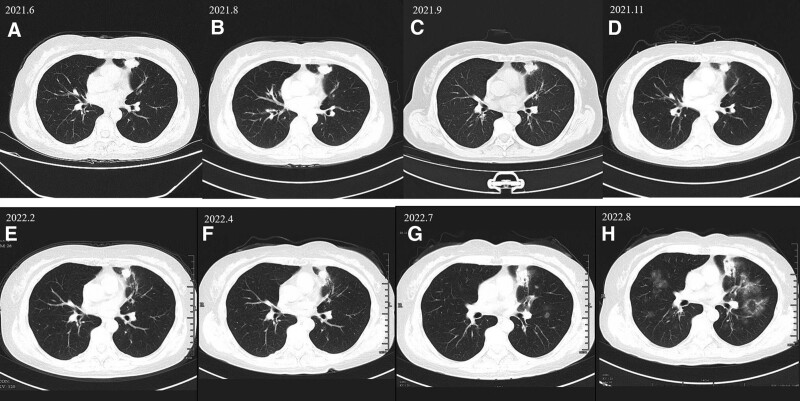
Results of chest CT scans before and after ICI treatment in a patient with cervical adenocarcinoma. (A) 2021.6.16. Tumor size: 25 × 20 mm. (B) 2021.8.3. The tumor measured 24 × 17 mm. (C) 2021.9.18. The tumor measured 23 × 17 mm. (D) 2021.11.26. The tumor measured 15 × 17 mm. (E) 2022.2.21. Tumor size:15 × 12 mm. (F) 2022.4.23. The tumor measured 15 × 13 mm. (G) 2022.7.16. The tumor measured 15 × 17 mm. (H) 2022.8.19. The tumor measured 15 × 17 mm. CT = computed tomography, ICI = immune checkpoint inhibitor.
